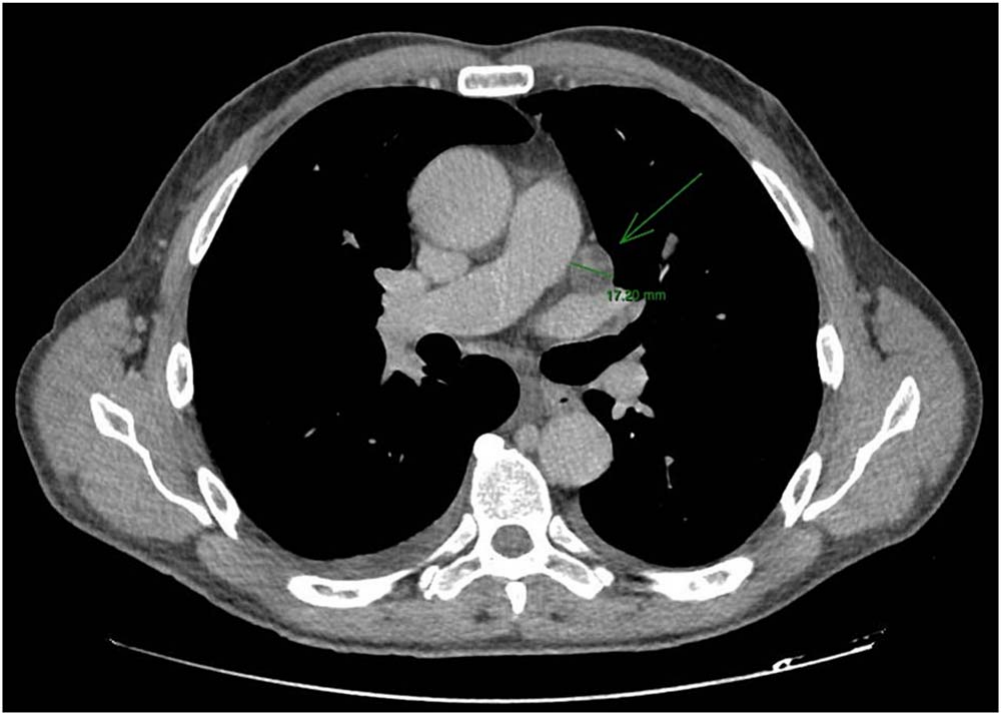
CT staging of the chest and pelvis showed the presence of subaortic, peri-esophageal, and hilar necrotic lymph node enlargement on the left, suggestive of secondary implants.
